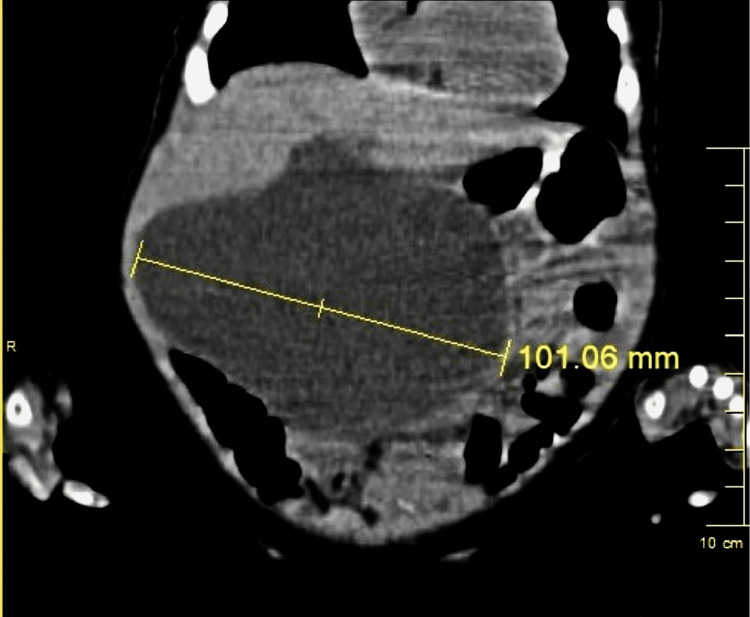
CT images of choledochal cyst measuring more than 10cm at its largest diameter CT: computed tomography
